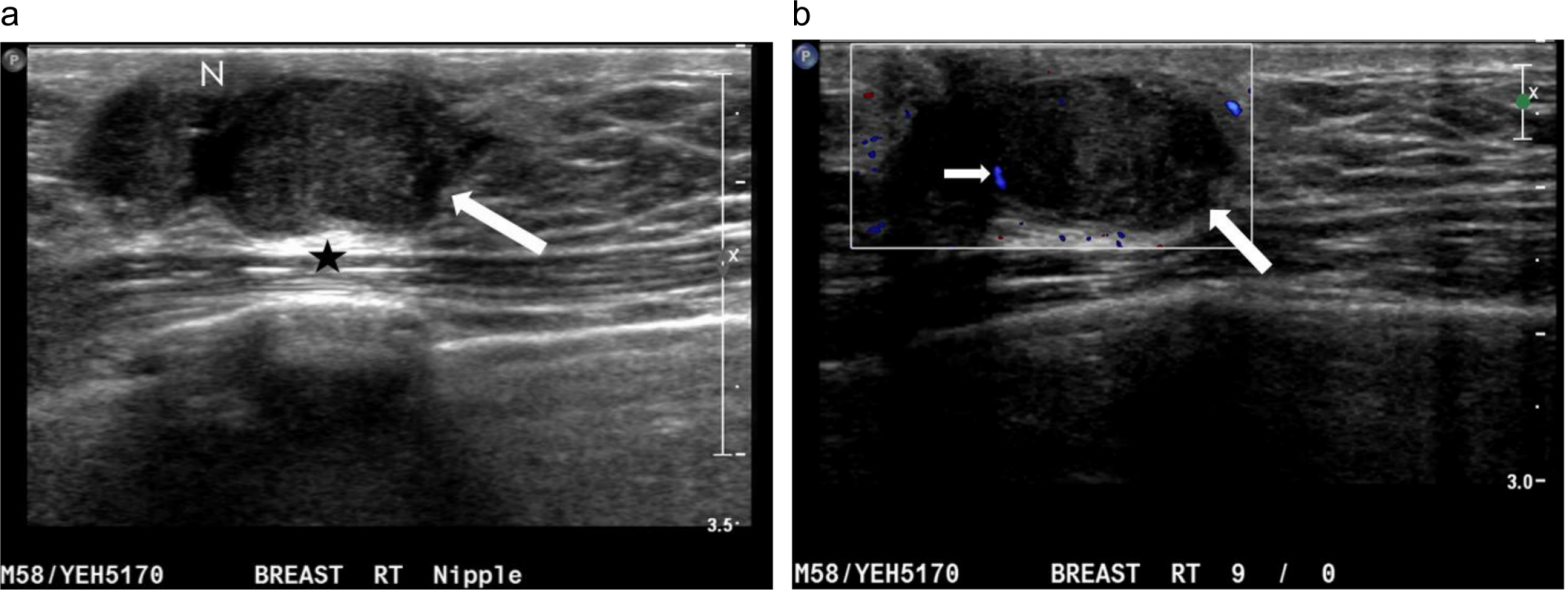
A palpable nodule in the right breast of a 58-year-old man. (a) Radial sonogram shows an irregular shape, a noncircumscribed margin, and a heterogeneously hypoechoic, subareolar nodule (arrow). The nodule has a parallel orientation and a mildly eccentric relationship to the nipple (N). There is posterior acoustic enhancement (black star). (b) Transverse color Doppler ultrasound shows a short color flow signal (short arrow) in the nodule (long arrow), categorized as low color flow with predominantly internal vascularity. The postoperative pathological diagnosis is invasive ductal carcinoma.
